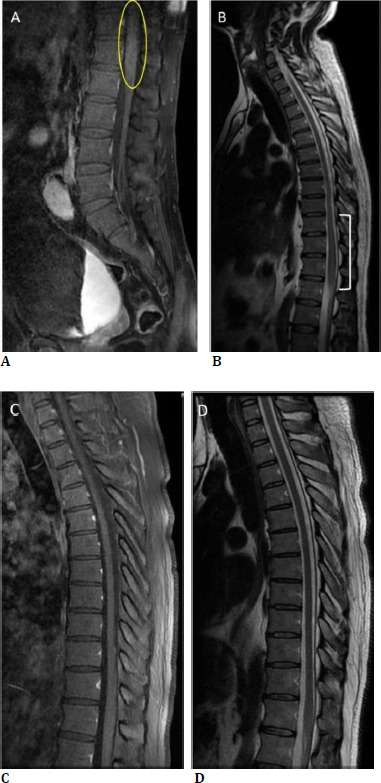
The patient’s first admission MRI images show enhancement (yellow circle) in the medulla spinalis on the sagittal contrast-enhanced T1-weighted image (A) and expansion (white line) in the medulla spinalis at the T8-12 levels on the T2-weighted image (B). After treatment, MRI images taken at 4 months appear normal on the sagittal contrast-enhanced T1-weighted image (C) and T2-weighted image (D)
